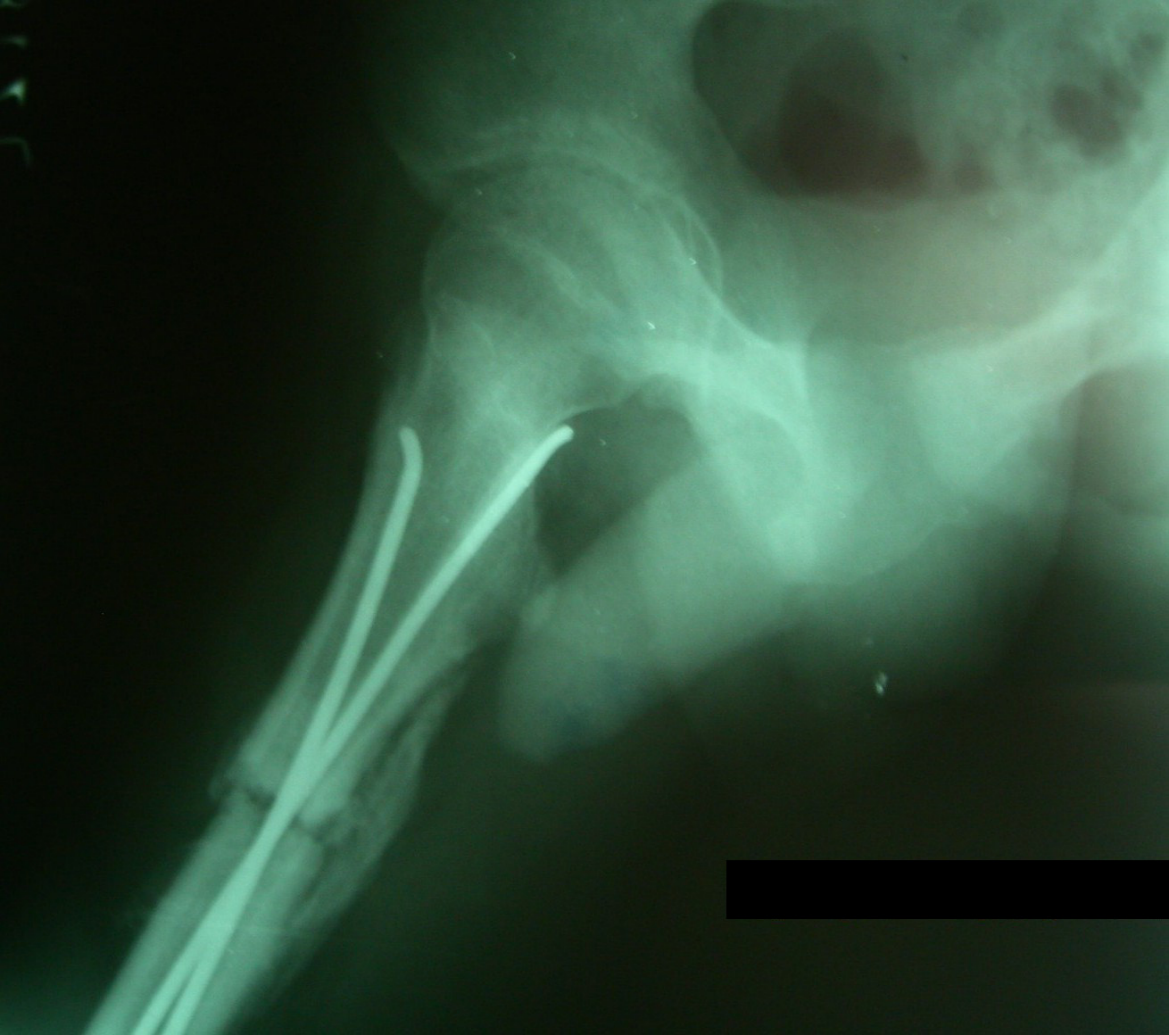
Lateral radiographs of patient in figure 5 at 5th postoperative week revealed penetration of posteromedial by medial nail, nail was retained till full union.
